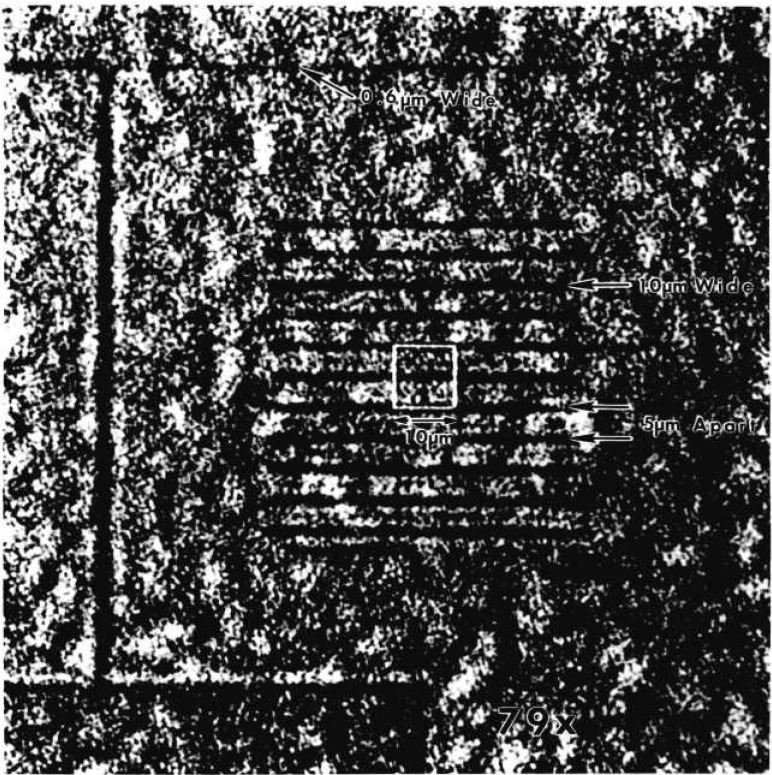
A radiographic image of a pattern of Pd lines on Si. This image was obtained with a magnification of 79 and a wavelength of 1.0 Å (12.3 keV). At this magnification, one CCD pixel represents 0.25 *μ*m.
